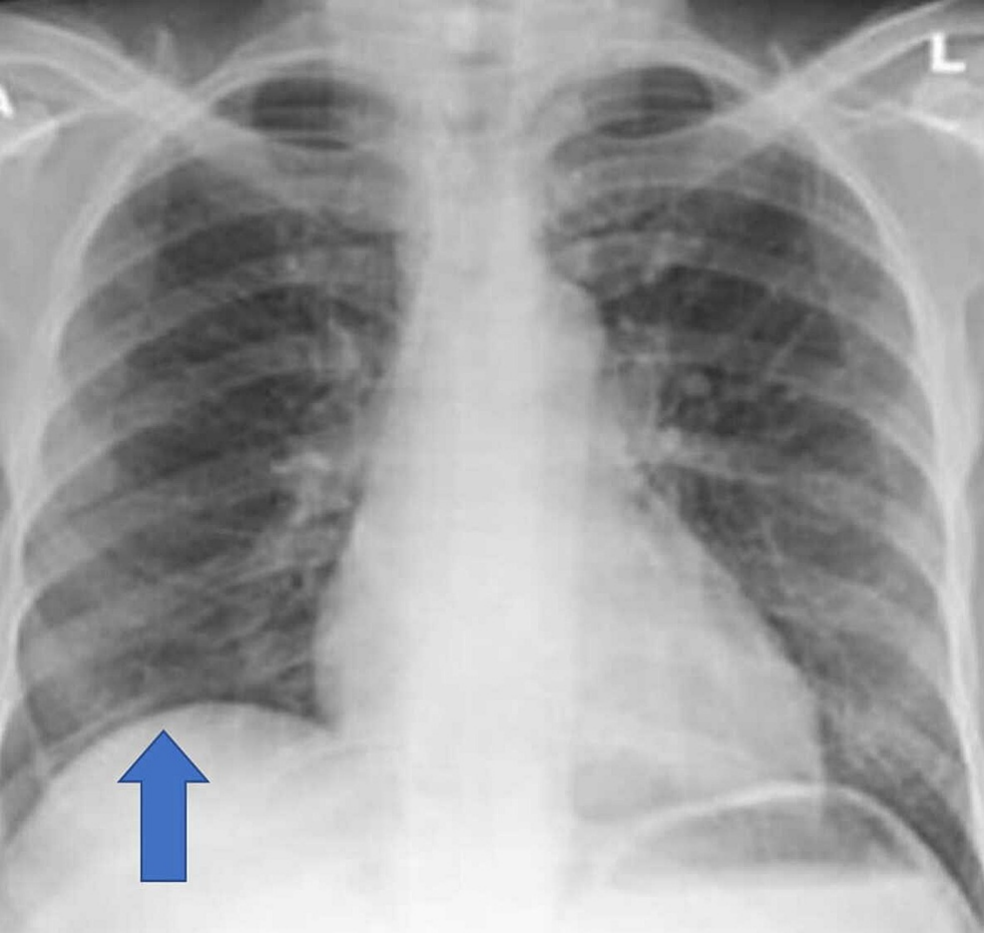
Chest radiograph shows resolution of the right-sided pleural effusion
